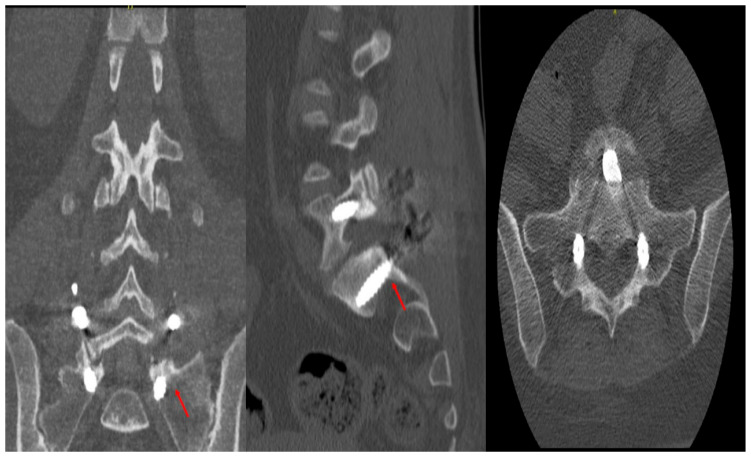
A four-grade system (A to E) was created to determine the pedicular screws’ position in a postoperative CT scan of the instrumented area. In this images, it is possible to see a Grade B medial breach antero-posterior, lateral, and axial views in the CT scan.
